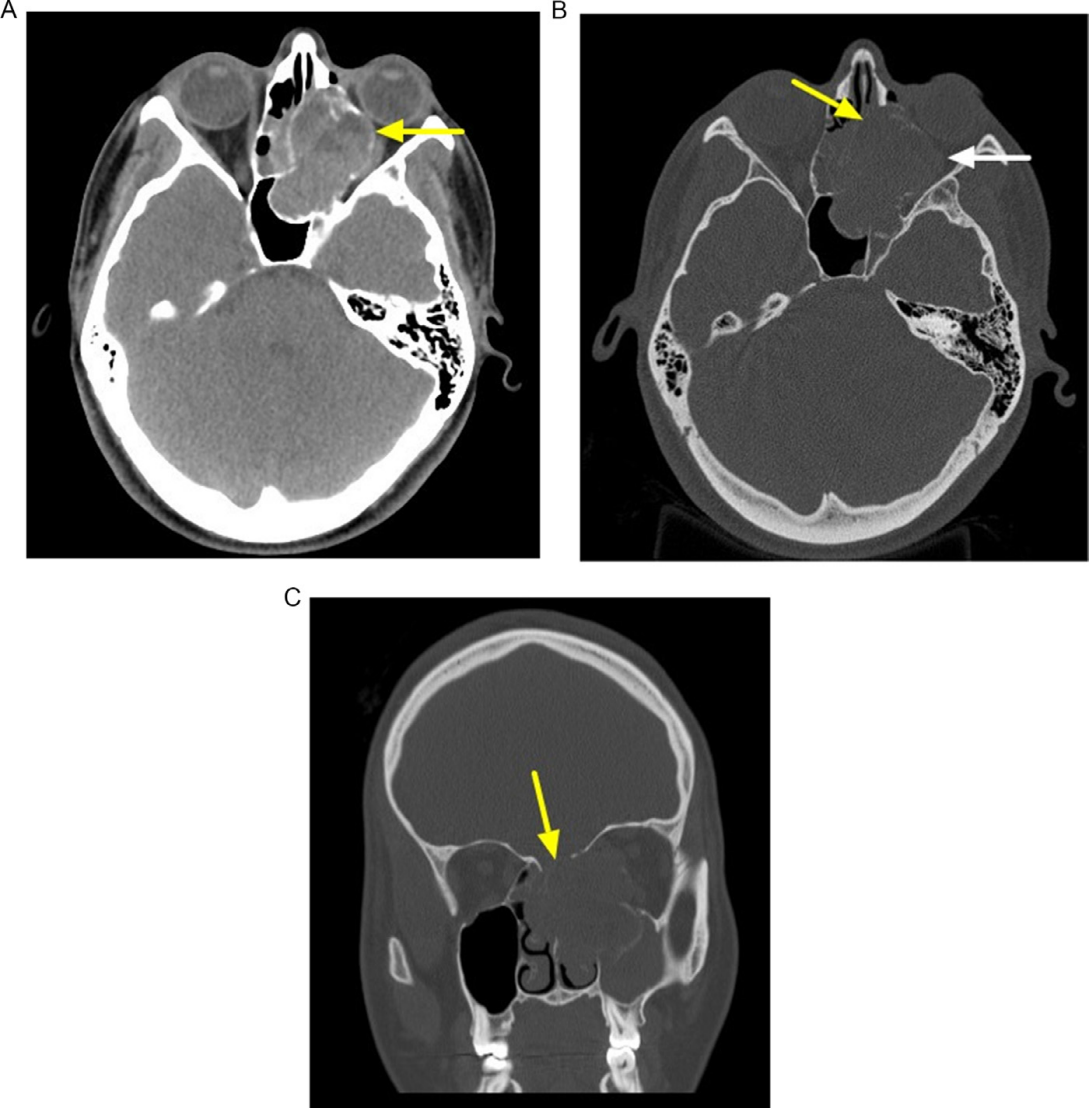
Nonenhanced CT of a paranasal sinus mass. (A) Axial soft tissue window demonstrates an expansile mass arising from the left nasal cavity with internal areas of hyperdensity and several apparent fluid-fluid levels (yellow arrow). Axial (B) and coronal (C) in bone window demonstrates multiple areas of bony expansion and marked thinning/erosion of the medial orbital wall (white arrow) and bony septum (yellow arrow) in Figure B and of the anterior skull base (yellow arrow) in Figure C. (Color version of figure is available online.)
